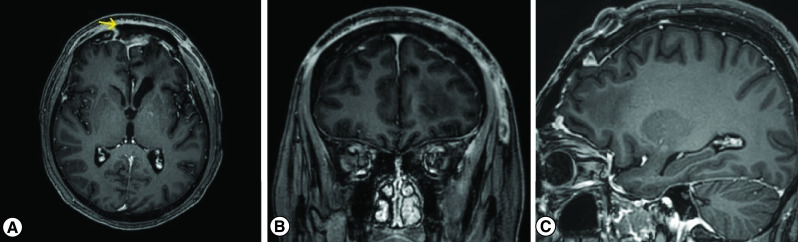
Postoperative MRI of the brain with contrast. (A) Axial, (B) Coronal and (C) Sagittal sequences showing en bloc tumor resection except the part inside the SSS with placing of an artificial bone-methylaacrylicacryltae (arrow). SSS: Superior sagittal sinus.
